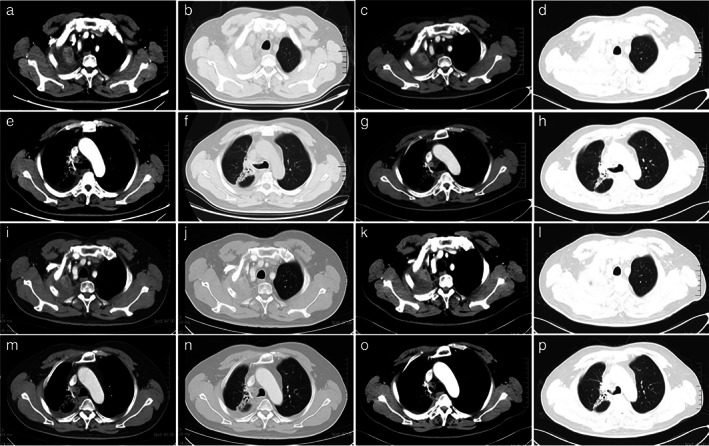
The CT images indicated a stable disease among March 10 (a, b, e, and f), June 10 (c, d, g, and h), September 16, 2021 (i, j, m, and n), and January 19, 2022 (k, l, o, and p)
